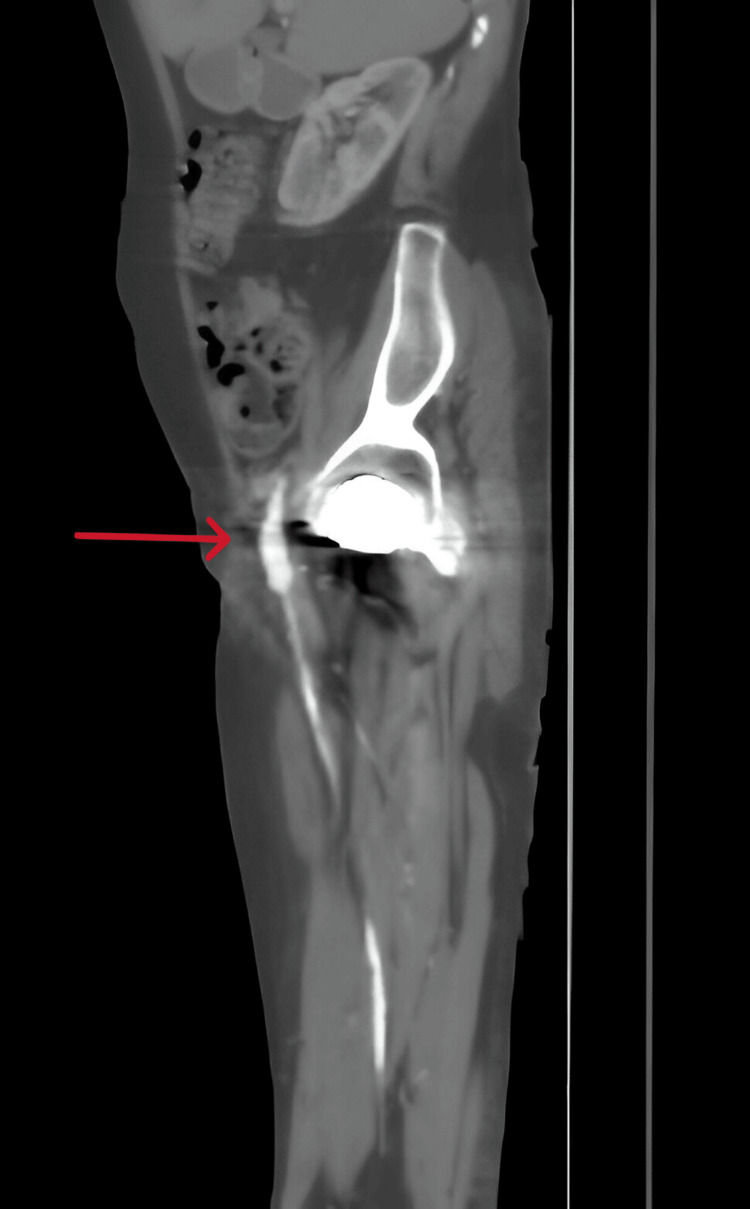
A follow-up CT scan showing resolution of the occlusion of the common femoral artery (red arrow).
